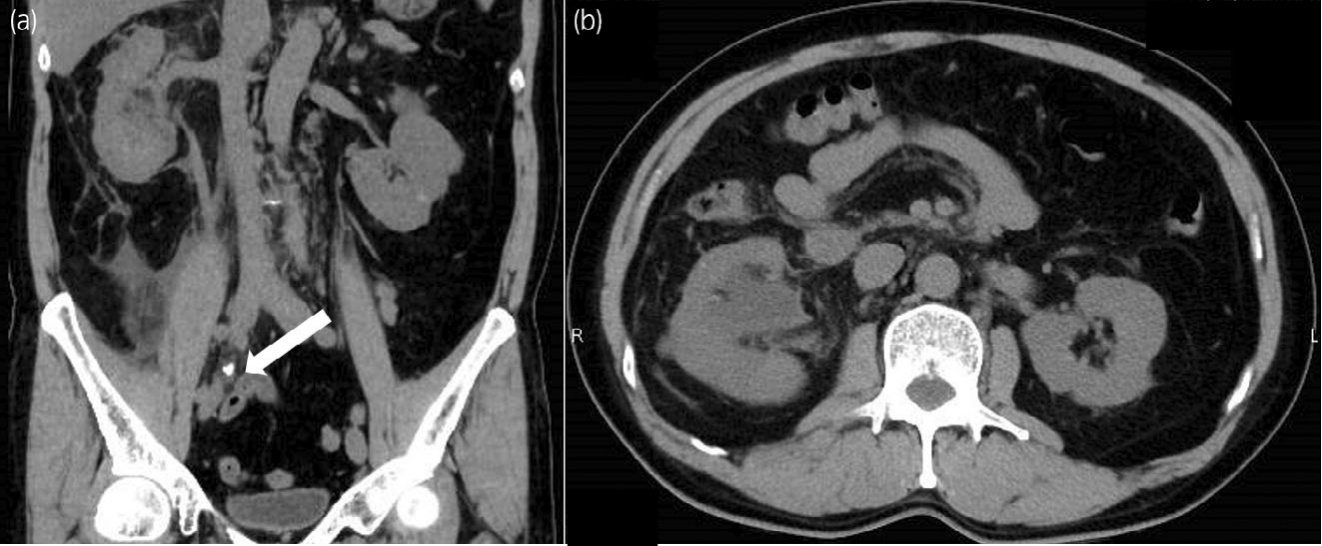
Non‐contrast CT at initial examination demonstrates an 8 mm diameter stone in the right middle ureter and hydronephrosis. (a) Coronal; (b) Axial.
